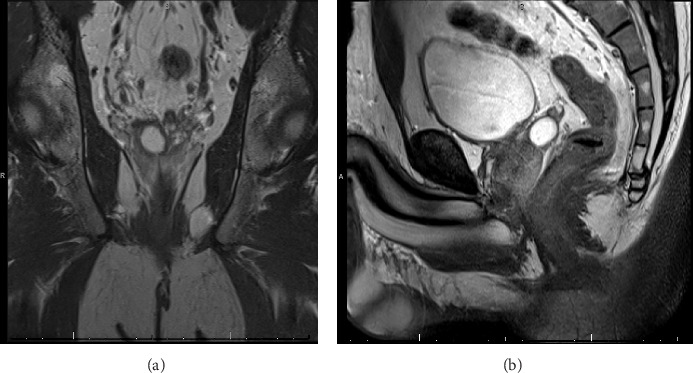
MRI of the prostate reveals right seminal vesicle cyst and low signal intensity. (A) Coronal view of the T2-weighted scan. (B) Sagittal view of the T2-weighted scan.
